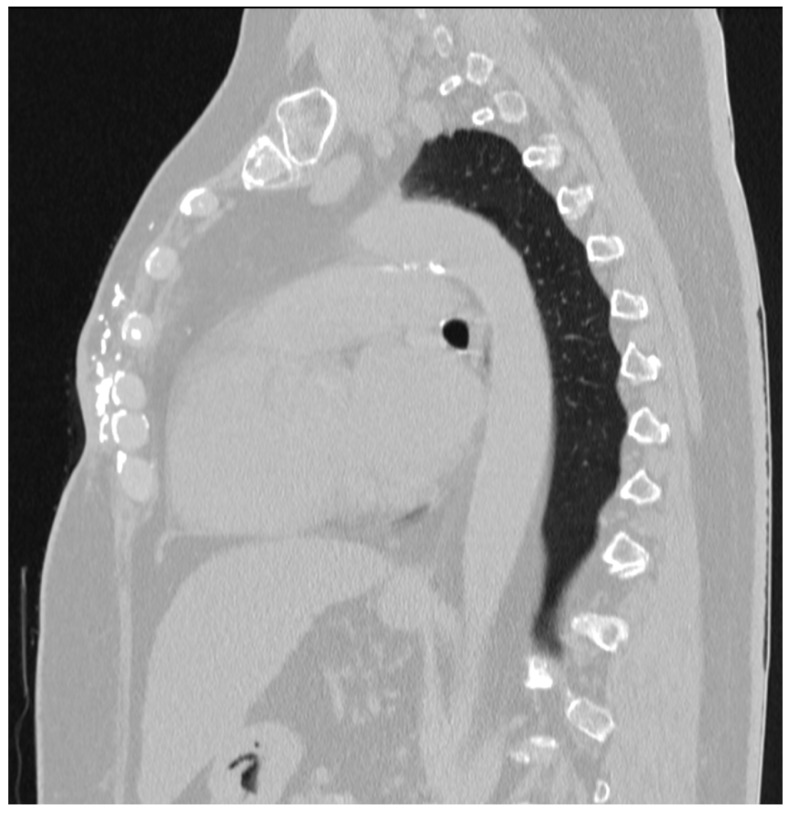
CT scan; multiple calcifications in the wound after the mastectomy ventrally to the sternum.
